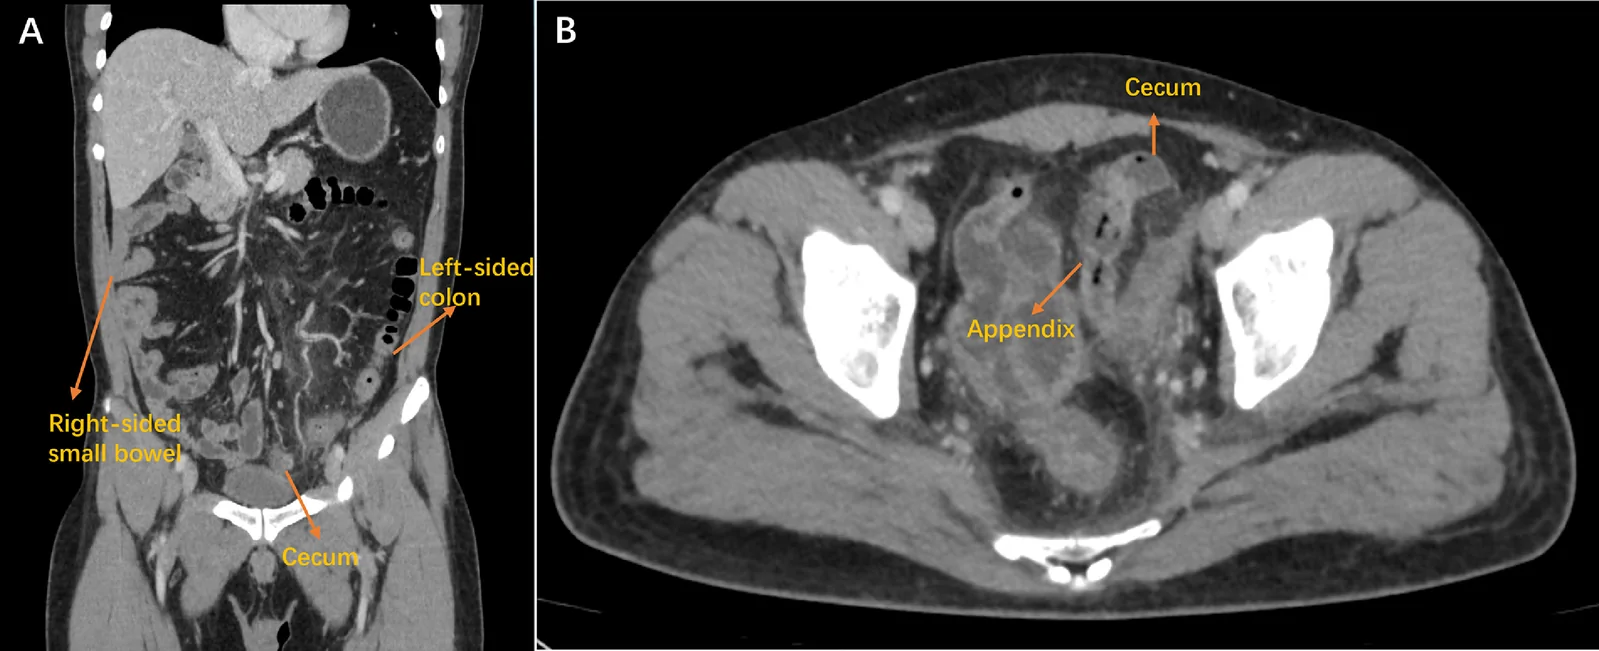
CT demonstration of intestinal nonrotation and the left-sided appendix. (A) Coronal multiplanar reconstruction demonstrates predominantly right-sided small bowel and left-sided colon, with the cecum located in the left lower abdomen. (B) Axial image demonstrates the appendiceal base arising from the malpositioned cecum, and the course of the blind-ending appendix in the left lower quadrant.
